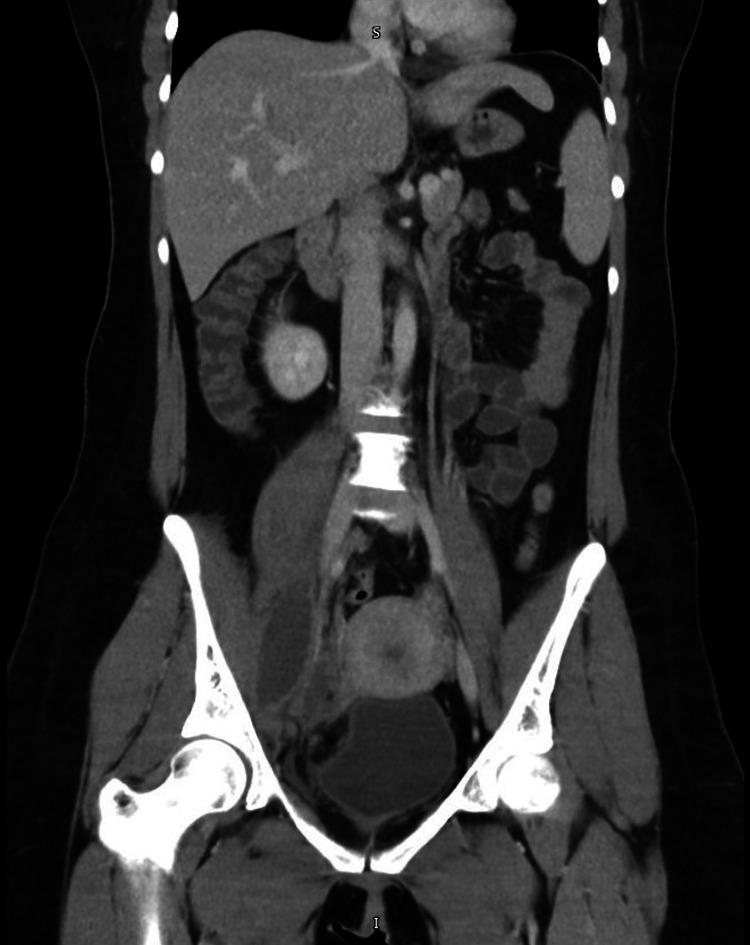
A CT scan of the abdomen and pelvis with IV contrast demonstrates a right lower quadrant inflammatory process and a right iliopsoas abscess.
